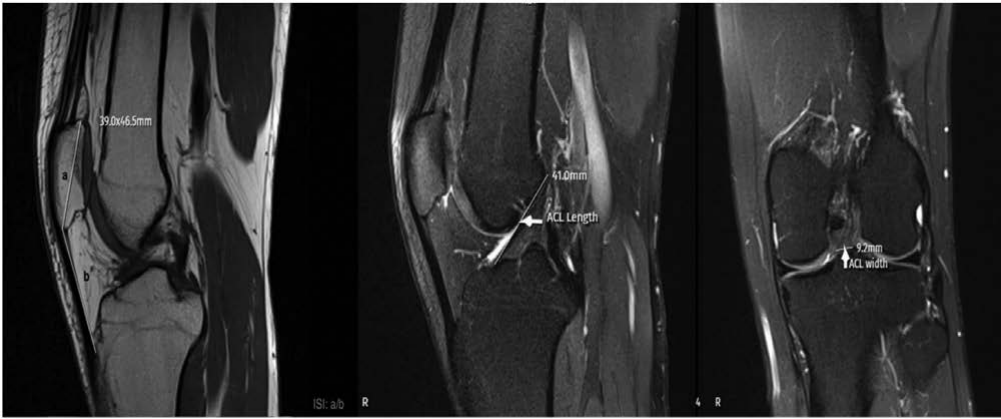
MRI measurement techniques of ISI, ACLL, and ACLW. ACLL = anterior cruciate ligament length, ACLW = anterior cruciate ligament width, ISI = Insall–Salvati index, MRI = magnetic resonance imaging.
